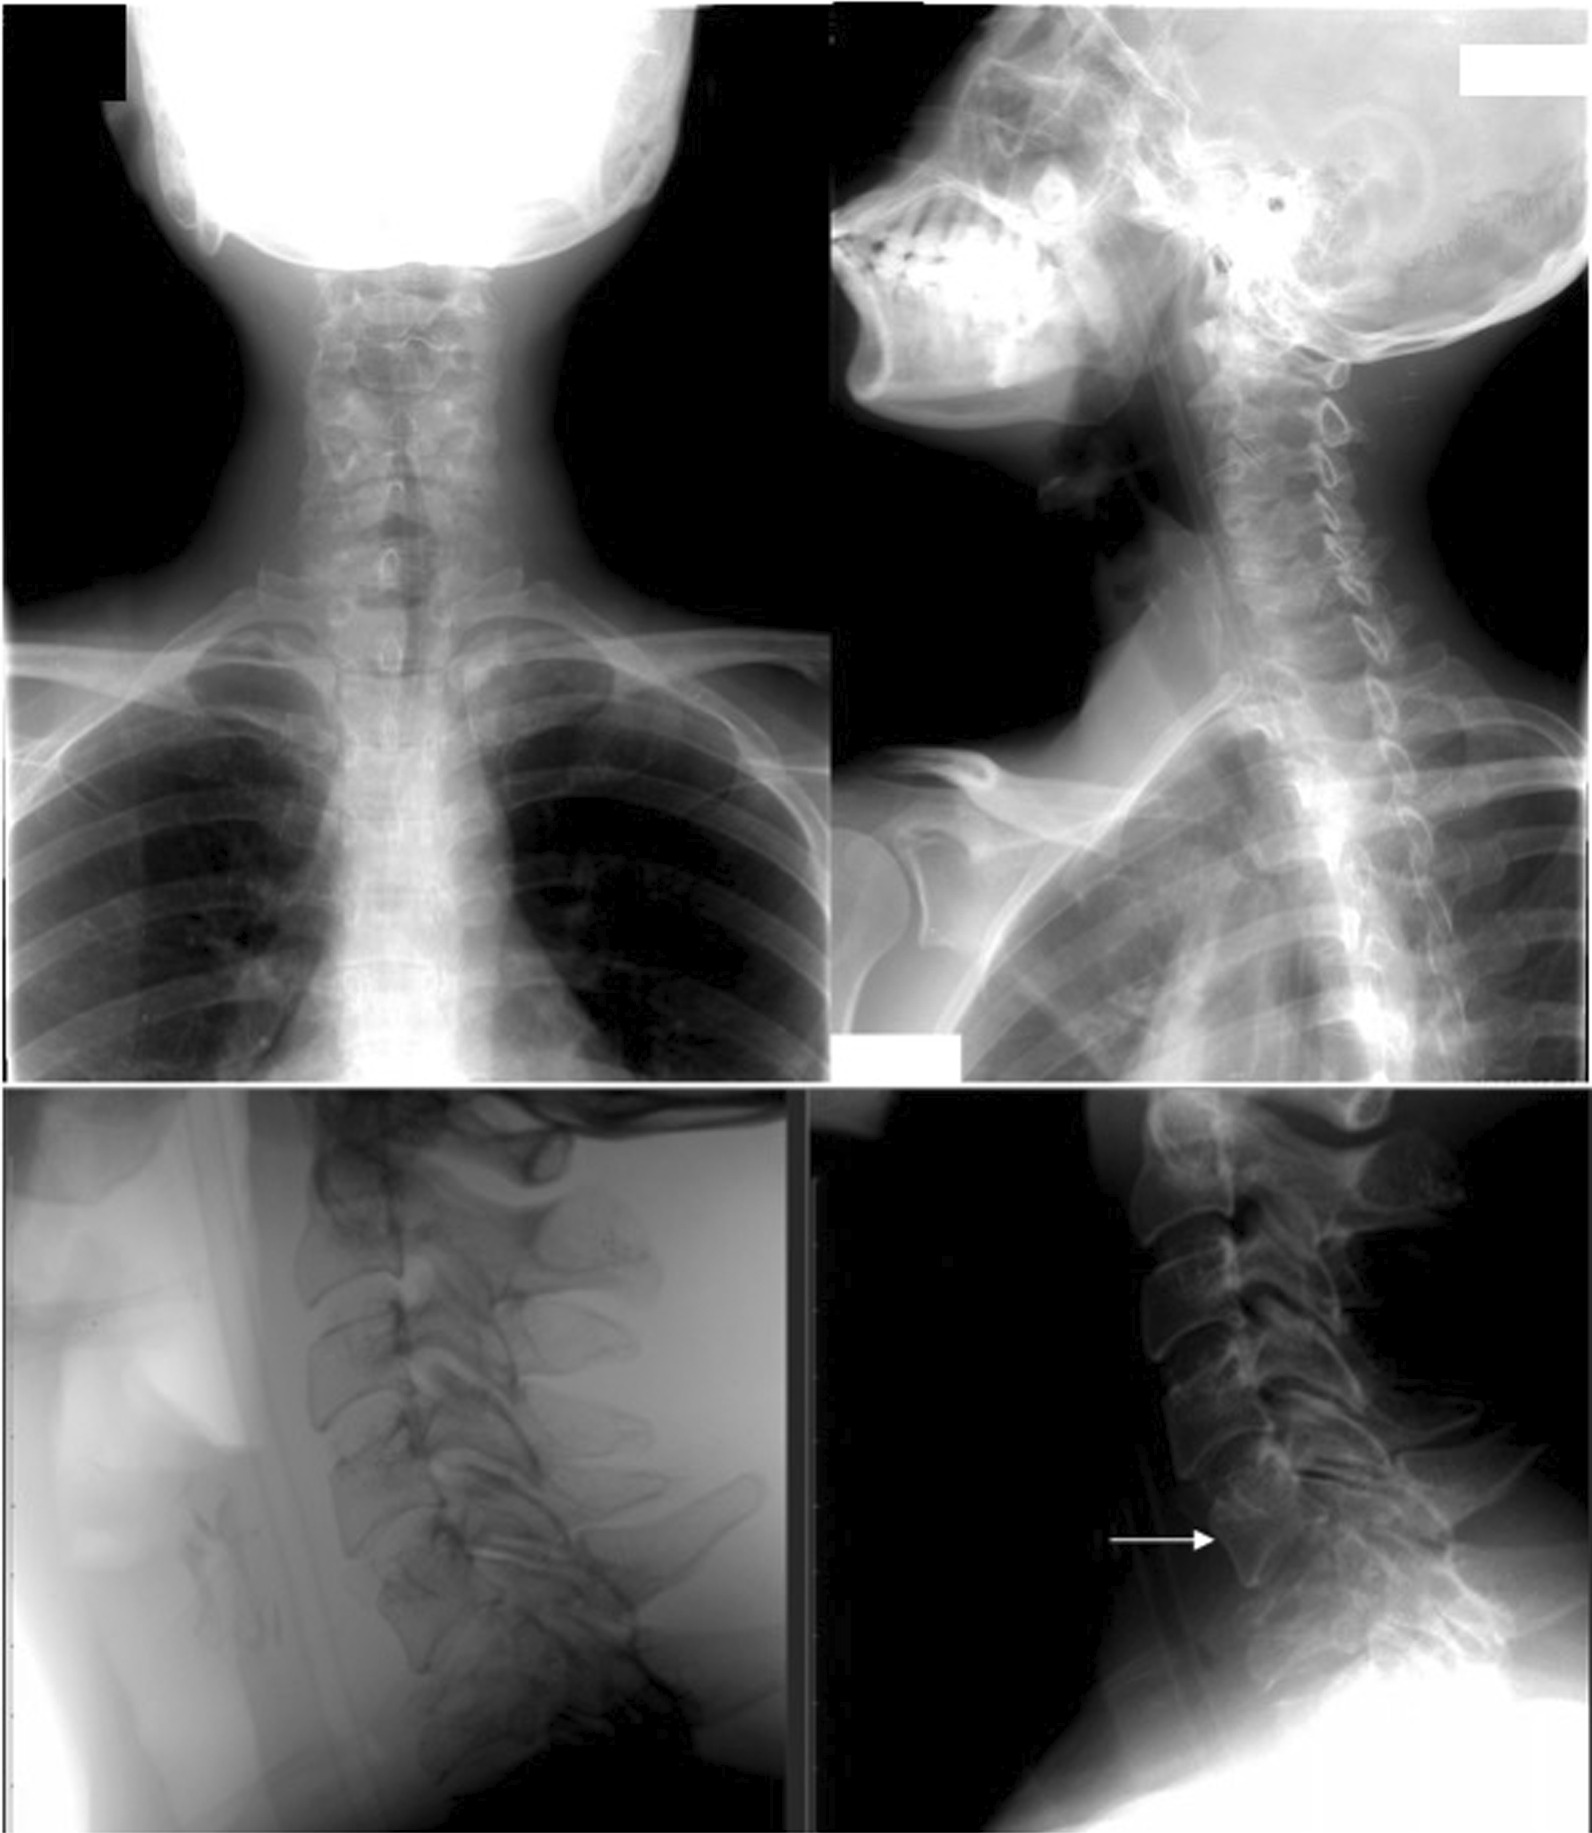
Plain cervical X-ray. The arrow shows burst fracture of the 6th cervical vertebra
